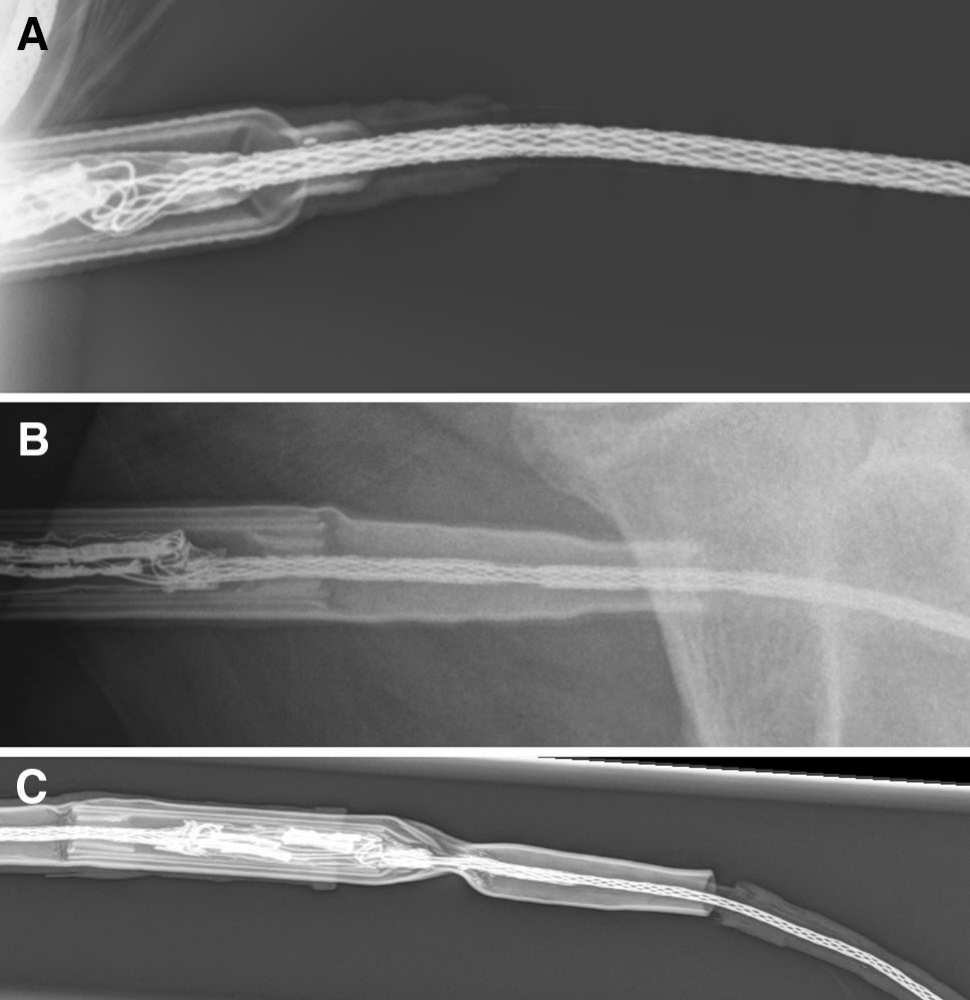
X-ray of the exchanged driveline after a 606 days, b 1977 days, and c 2801 days
